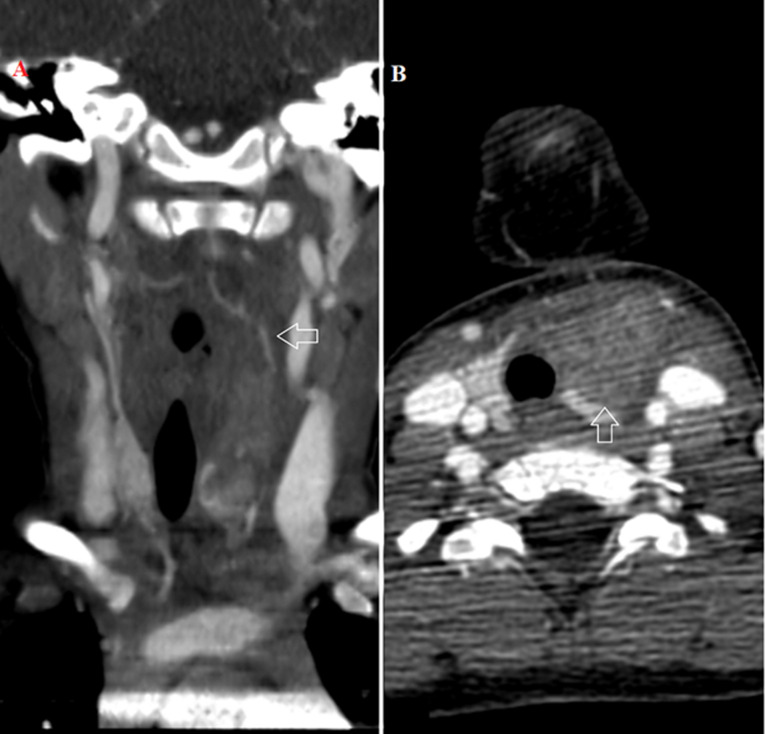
computed tomography with intravenous contrast medium: A) axial imaging heterogeneity in the left thyroid lobe that corresponds to an abscess, the left carotid artery, and internal jugular vein are patent; B) coronal imaging, the inflammatory lesion extends inferior to the hyoid bone to the thoracic inlet, anterior mediastinum fat planes are intact, there is a mild right tracheal deviation
